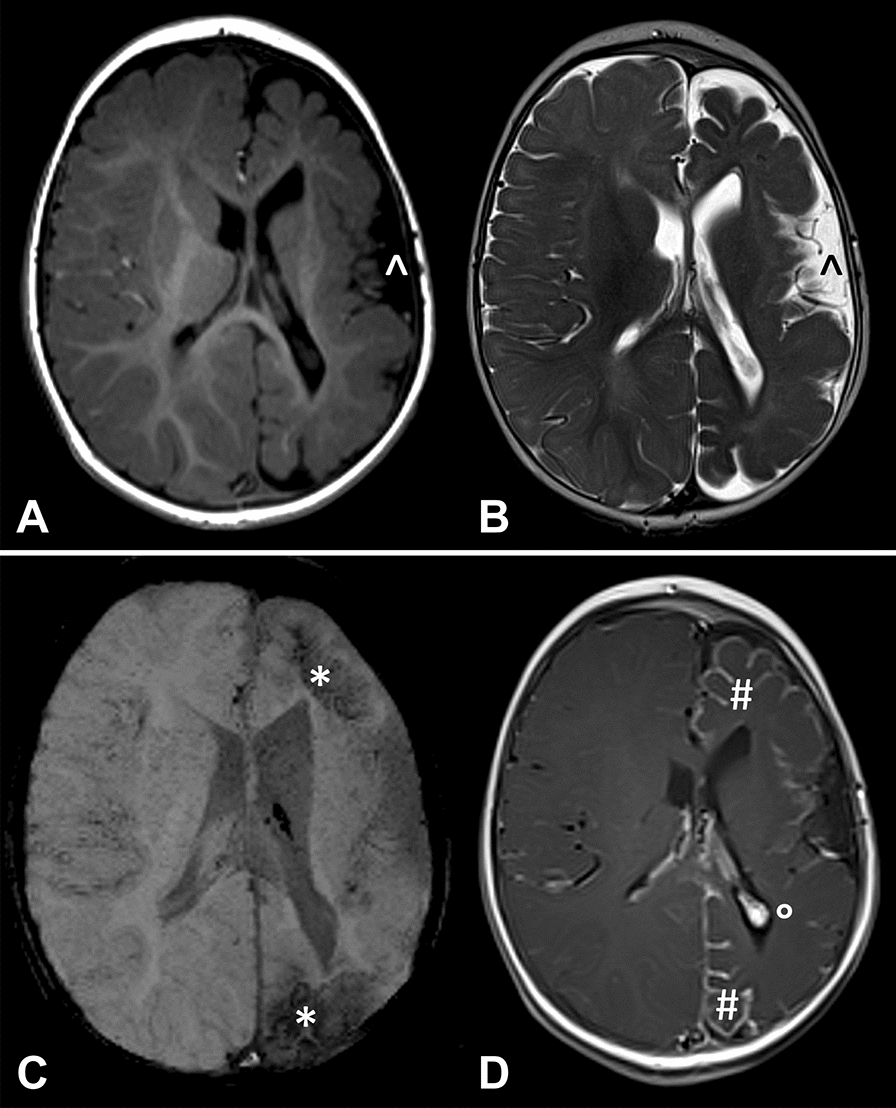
Magnetic resonance imaging (MRI) of the brain in a child with Sturge–Weber syndrome. Axial T1 (A), T2 (B), susceptibility- (C), and gadolinium-enhanced T1 (D)-weighted images. Note fronto-parietal atrophy of the left hemisphere with enlargement of adjacent subarachnoid spaces (^); the sharp hypointensity on susceptibility-weighted imaging sequence corresponds to meningeal calcification (*). Pial enhancement over the left frontal and parietal lobes (#), as well as hypertrophied choroid glomus (°) are visible
